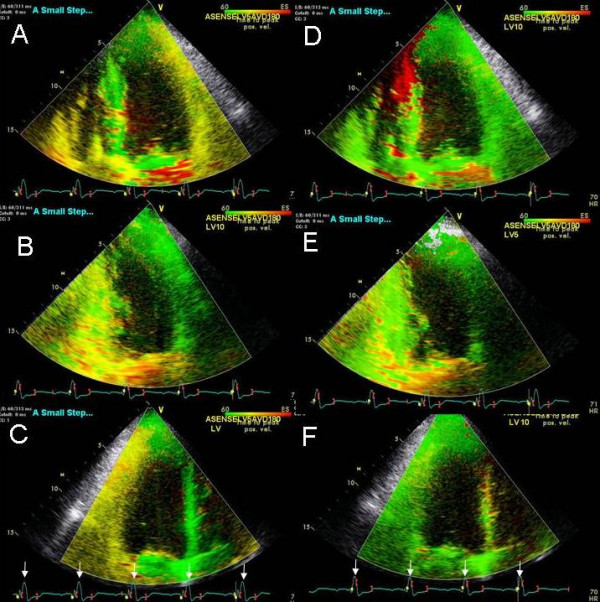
Panels A, B and C show TSI in the apical 4 (A), 2 (B) and 3 chamber (C) views with LV pre-excitation of 5 ms and panels D-F are corresponding views with LV pre-excitation of 10 ms in a 67 year old male with non ischemic cardiomyopathy post CRT. Note improvement in lateral, right ventricular free wall, inferior and posterior wall delay with LV pre-excitation of 10 ms. Some delay in interventricular septal contraction (red in distal septum in panel D and yellow in anterior septum in panel F) occurred with LV pre-excitation of 10 ms. Also note the narrowing of QRS complex with LV pre-excitation (white arrows C and F).
